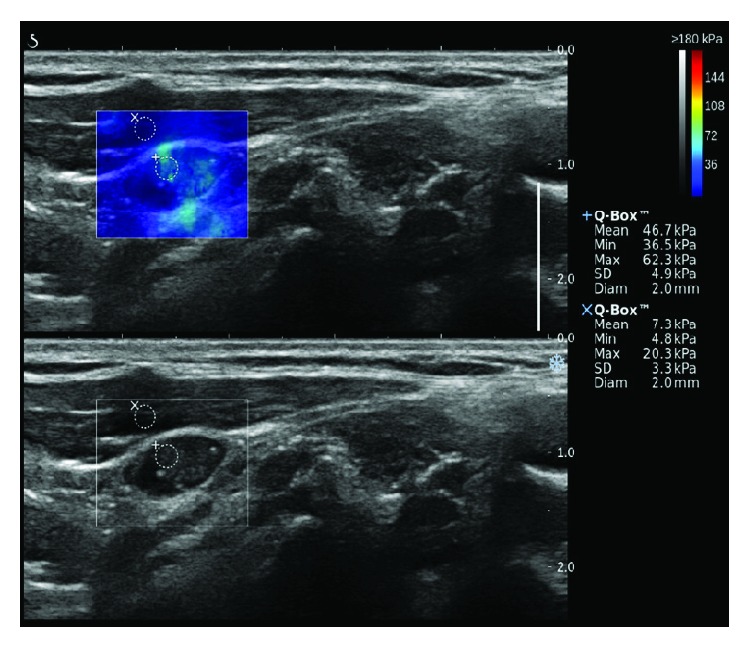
Shear-wave elastography (upper image) and gray-scale ultrasound (lower image) of metastatic cervical lymph nodes.
